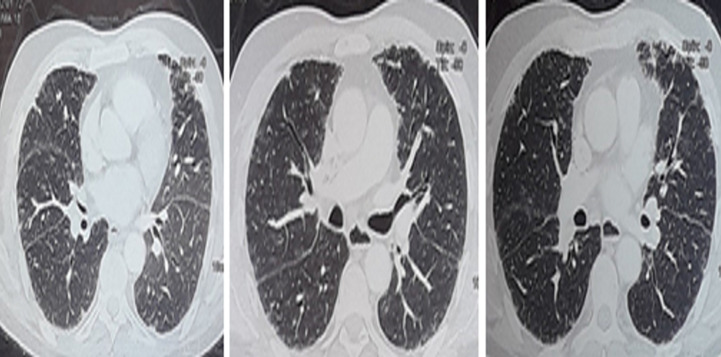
chest CT showed traction bronchiectasis with peripheral reticulations in the upper lobes
